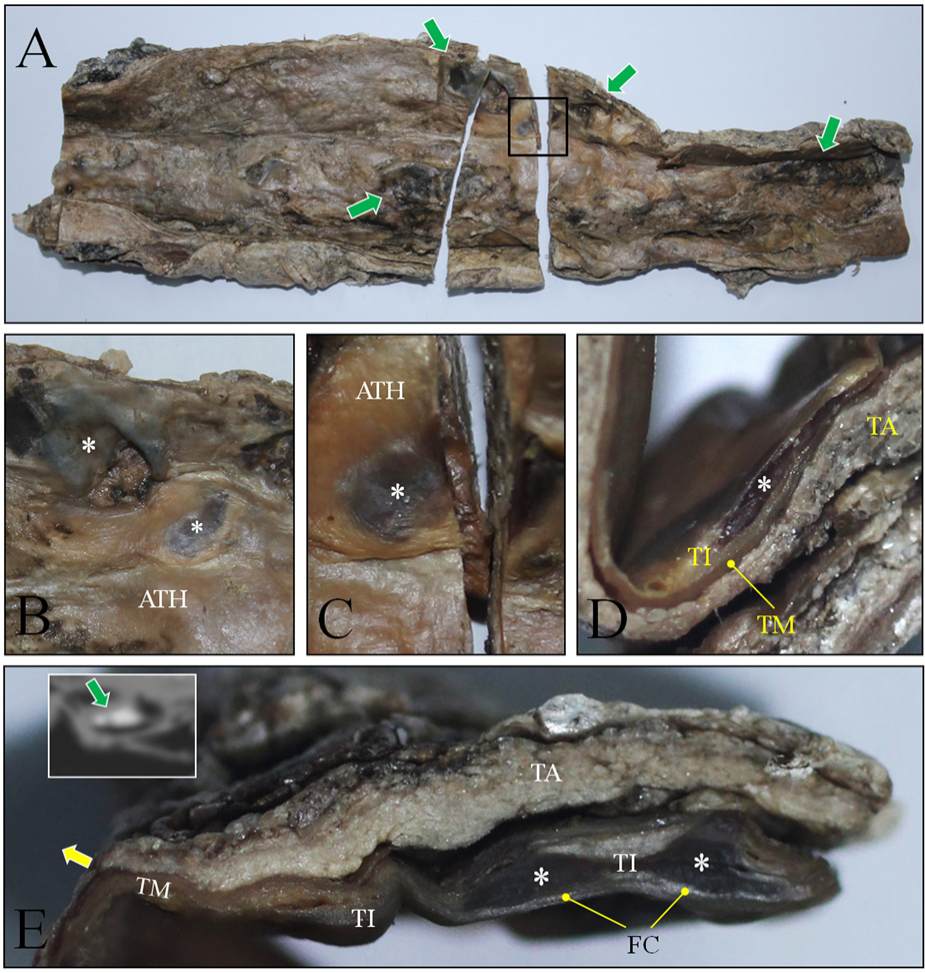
Cross-section of abdominal aorta. (A) Longitudinal section was done on the anterior wall to expose the inside of aorta. Many atheromatous plaques (indicated by green arrows) were seen on the aorta wall. (B) and (C) Magnified image of fully developed atheromatous plaque (ATH) Asterisks indicate the area where necrotic center is present beneath the fibrous cap. (D) Cross-section view of atheroma in (C). Asterisk indicate the same necrotic center seen in (C). TI, TM and TA are tunica intima, tunica media and tunica adventitia, respectively. (E) Magnified image of another atheroma. Asterisks indicate necrotic centers beneath the fibrous cap (FC). Inset is the CT image of aorta. The level of CT image is similar to that of cross-section (E). Green arrow indicates calcifications in aorta wall. They correspond to the necrotic centers seen in cross-section (E).
